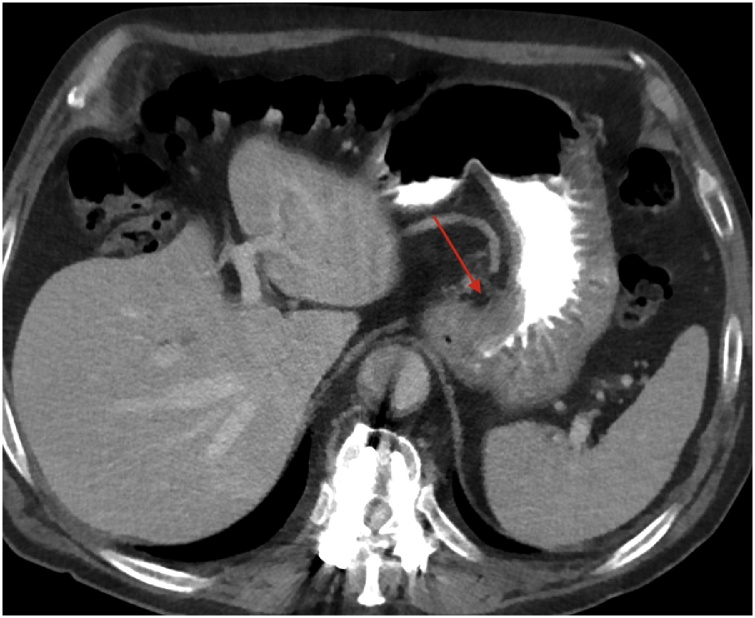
Axial CT venous phase (January 2016) demonstrated the irregular wall thickening of the subcardial lesser curvature (arrow) referable to the primary gastric neoplasm.
